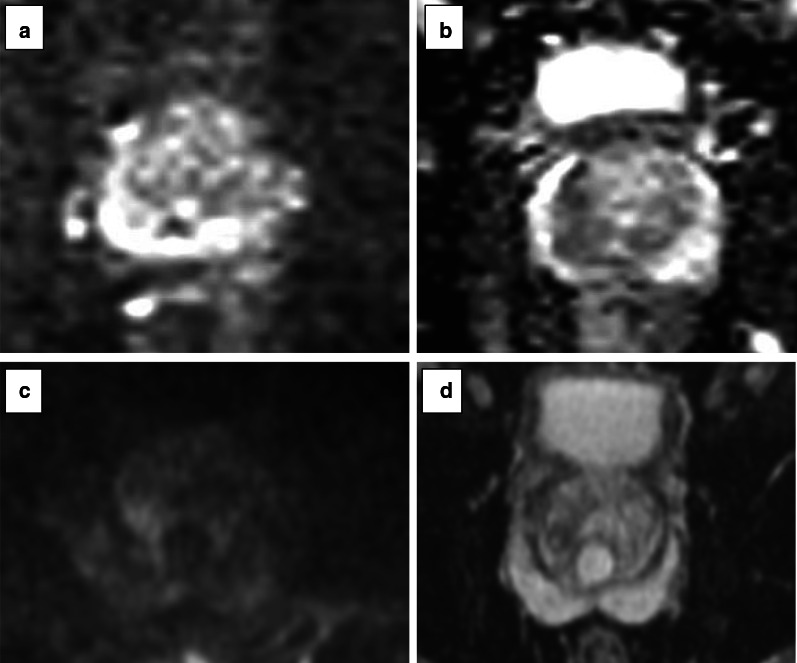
DWI of suboptimal (a, b) and optimal (c, d) in-plane resolution for the high b sequence (a, c) and ADC map (b, d), respectively
